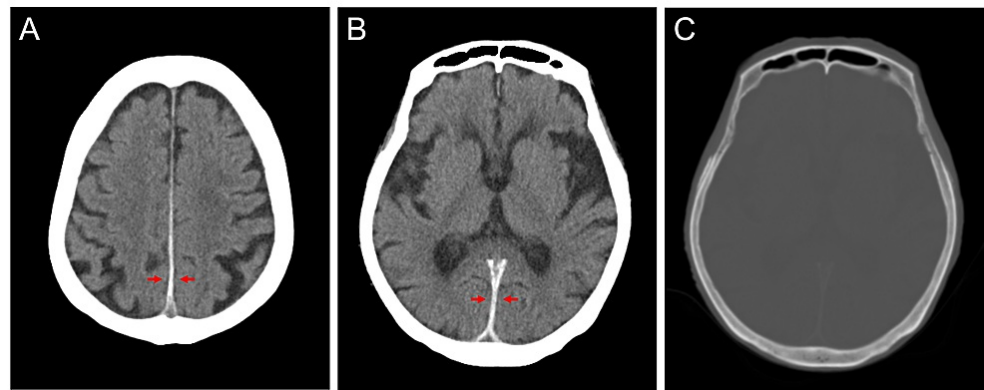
Axial images of non-contrast CT scan of the brain. CT images of the brain show a slight increase in the thickness and a high increase in the density of the posterior superior (A, arrows) and inferior (B, arrows) parts of the falx cerebri. The bone window of the same image (B) shows no calcification of the falx (C).
